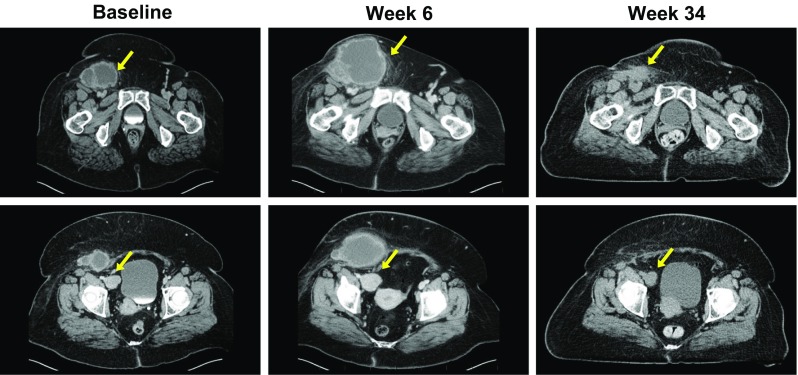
Example of a patient treated with T-VEC with a durable response and PPR due to enlargement of existing lesions. The patient had recurrent stage IVM1c melanoma of the right lower extremity with multiple regional and distant nodal metastases and elevated serum lactic dehydrogenase. Right inguinal and iliac lymph nodes were injected with T-VEC under ultrasound guidance (from week 14 for the iliac lymph node). PPR and increase in size in both injected and uninjected lesions were documented during the first 26 weeks of treatment. Responses are reported per External Assessment Committee. Titles above each photography are weeks on study. Partial response was recorded on week 40, and continued for 28 weeks. *PPR* progression prior to response
